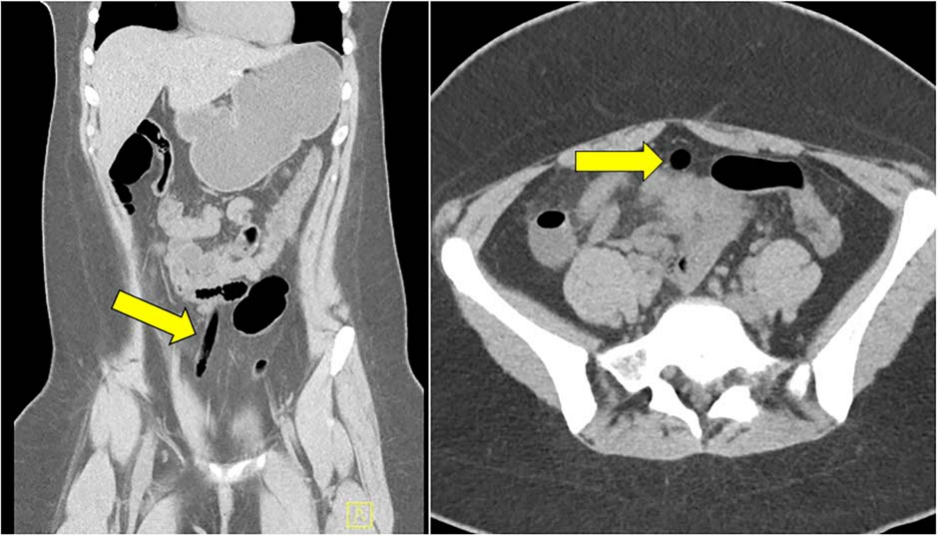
CT scan of abdomen and pelvis showing the presence of an air-filled foreign body in coronal and axial view.
